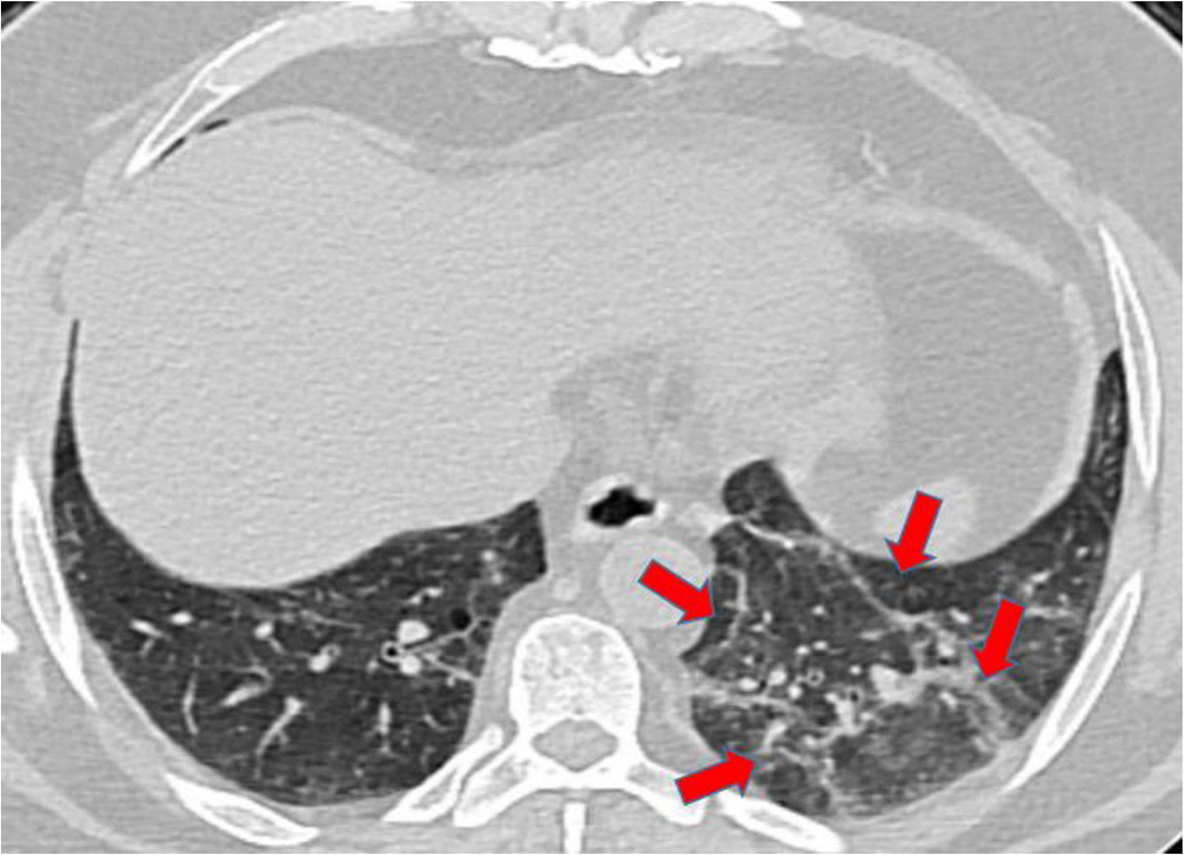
A 52-year-old male patient who had positive contact history with recently proved COVID-19 patient and was completely asymptomatic: Axial HRCT chest lung window showed left lower lobar patchy GGOs with secondary fibrosing changes (red arrows). Clinical decision was home isolation, medical treatment, and PCR testing. Positive PCR result for COVID-19 was proved later
